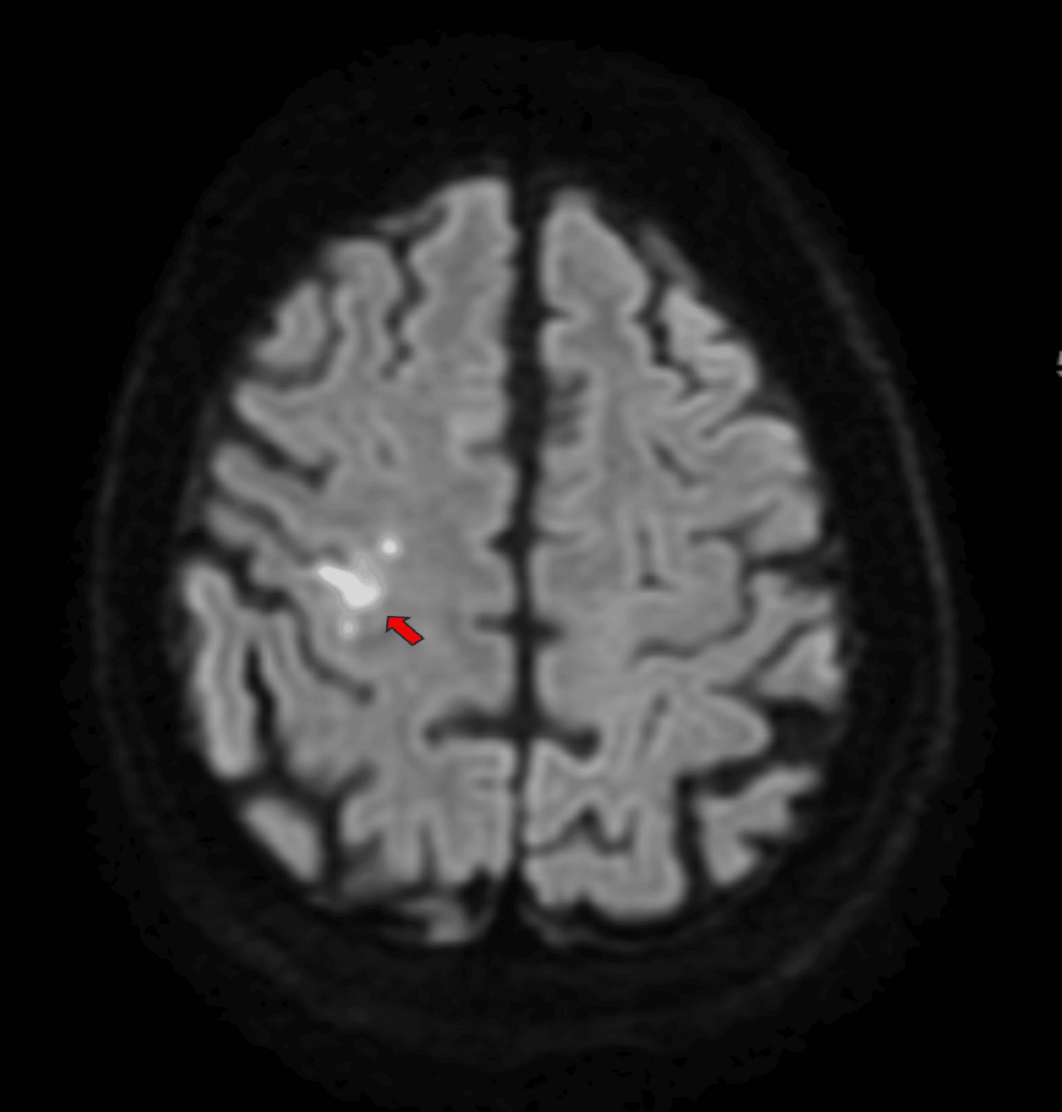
MRI of the brain showing a small acute infarct in the right precentral gyrus, specifically involving the hand knob area. There are a number of small regions of restricted diffusion on the right-hand knob area on a background of small vessel disease.
